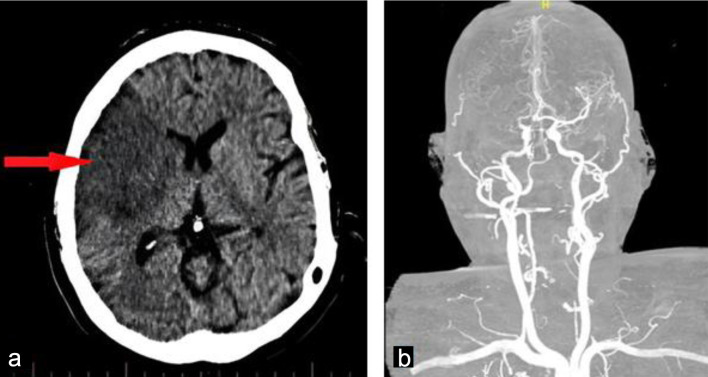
Admission brain computed tomography without contrast obtained on admission showing a well-defined hypodense lesion in the right (arrow), consistent with an acute embolic ischemic infarction (a). Cerebral angiography showed occlusion of the right middle cerebral artery (b).
